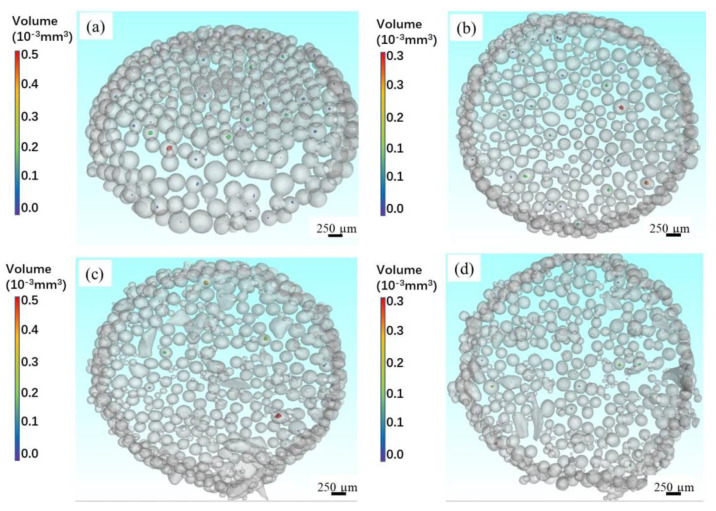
The 3D reconstructed CT of Ti-6Al-4V powders at (a) 8000 RPM, (b) 10,000 RPM, (c) 12,000 RPM, (d) 14,000 RPM.
